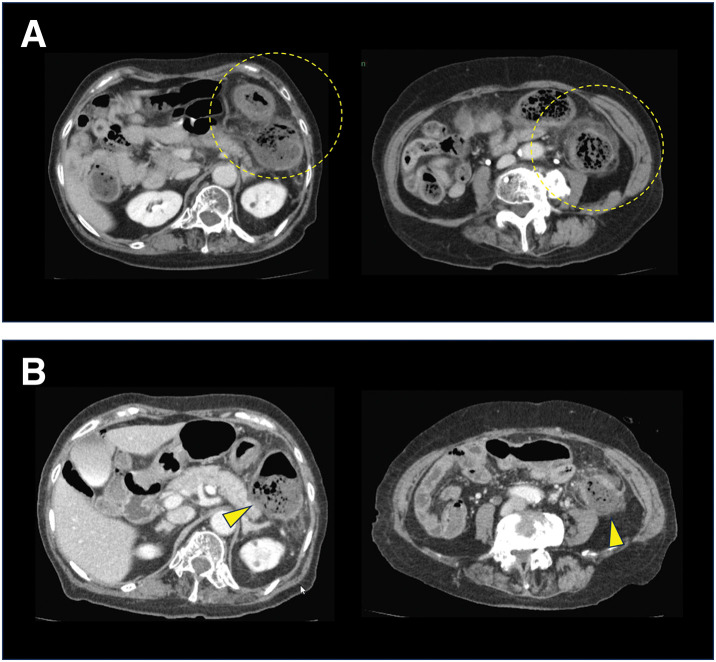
Contrast-enhanced CT scan images of the patient. (A) Contrast-enhanced CT scan images taken at the time of diagnosis of ischemic colitis. Edematous wall thickening and the dirty fat sign are observed in the splenic flexure and the descending colon (yellow circle). (B) Contrast-enhanced CT images taken at the time of progression to gangrenous ischemic colitis. Contrast enhancement defects and thinning of the colonic wall are observed in the splenic flexure and descending colon (yellow arrowhead).
